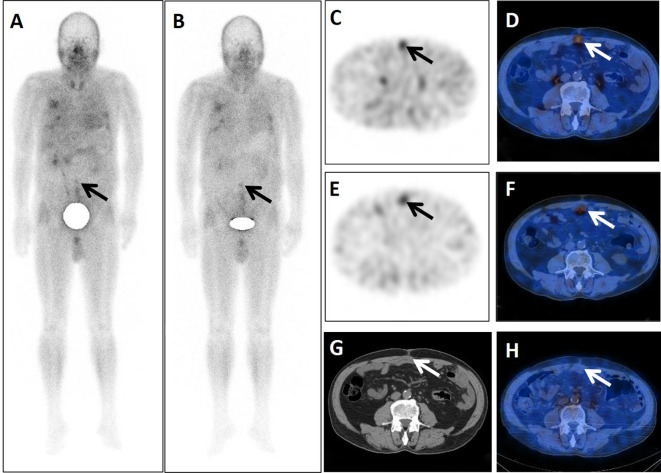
A 71-year-old postoperative male patient with a tumor in the sigmoid colon underwent dual-phase ^99m^Tc-FAPI-04 SPECT/CT. The early anterior whole-body MIP image (A) showed abnormal accumulation of radioactivity in the middle lower abdomen (arrow). The corresponding axial SPECT image (C, arrow) and fused ^99m^Tc-FAPI-04 SPECT/CT image (D, arrow) showed ^99m^Tc-FAPI-04 uptake in the lesion with TBR = 3.10. The lesions were dimmer in the delayed anterior whole-body maximal intensity projection (MIP) image (B, arrow), corresponding axial SPECT image (E, arrow), and fused ^99m^Tc-FAPI-04 SPECT/CT image (F, arrow) with TBR = 2.84. The axial CT image (G) showed an irregular postoperative scar measuring 48 mm × 21 mm (arrow). Six months later, this scar did not take up ^99m^Tc-FAPI-04 abnormally (H, arrow).
